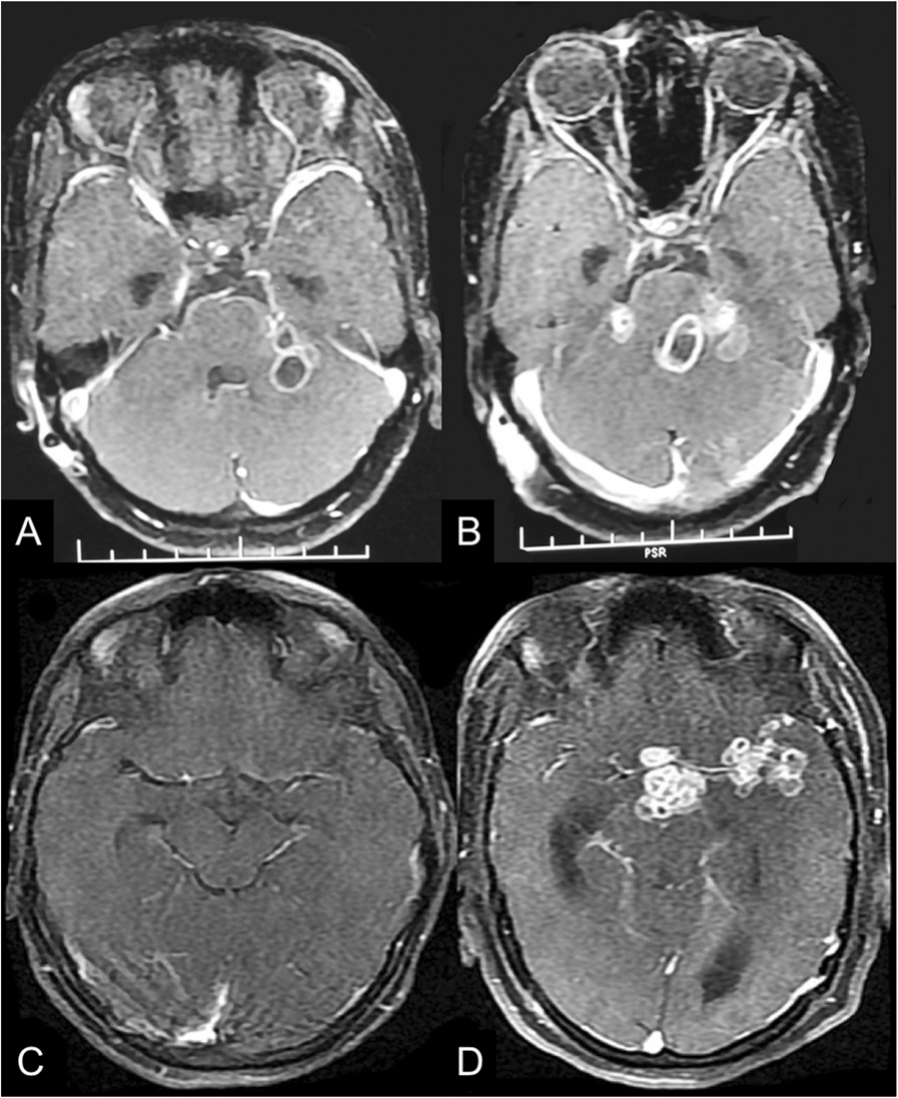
T1-weighted Gadolinium-enhanced MRI (axial sections) of the brain of a patient with TBM depict the development of additional (paradoxical) tuberculoma (Baseline-a, at 5th month-b) at the pontocerebellar level. Similar sequence in another patient shows development of paradoxical optochiasmatic arachnoiditis along with multiple tuberculomas (Baseline-c, at 3rd month-d)
